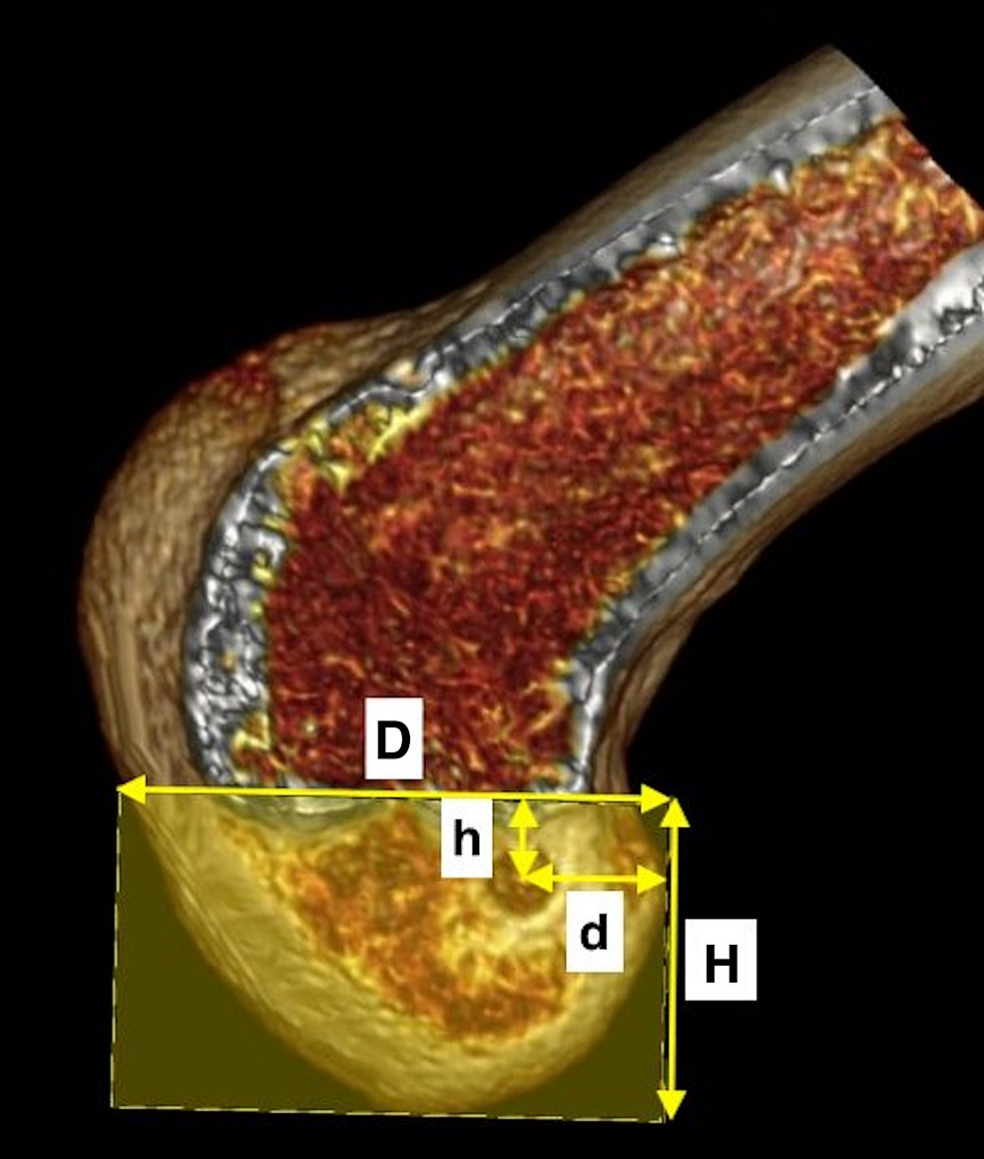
A representative three-dimensional computed tomography image of a distal femoral sagittal section through the Blumensaat line. The femoral tunnel entry aperture’s depth (d) is measured as a proportion of the rectangle’s overall anteroposterior extent (D) drawn using the quadrant method. Similarly, the femoral tunnel entry aperture’s height (h) is measured as a proportion of the rectangle’s overall superior-inferior extent (H).
